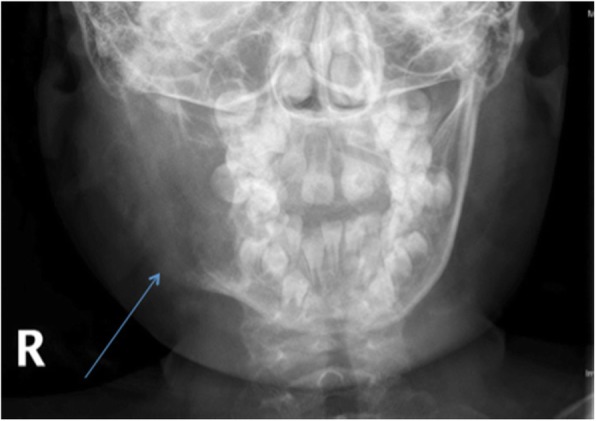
Frontal radiograph of the facial bones at initial presentation to the emergency department. Arrow is pointing to site of lesion
